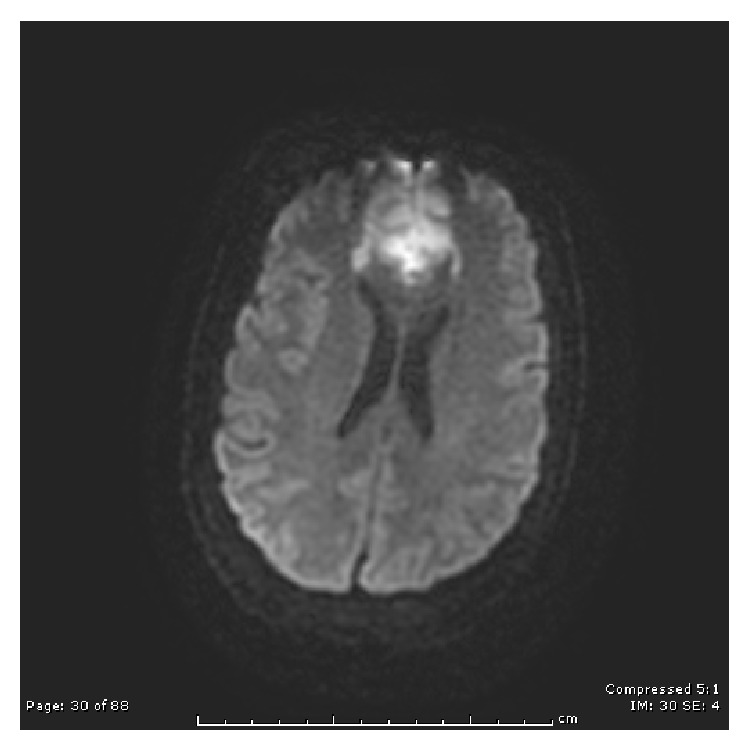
DWI MRI showing restricted diffusion bilateral ACA territory.
